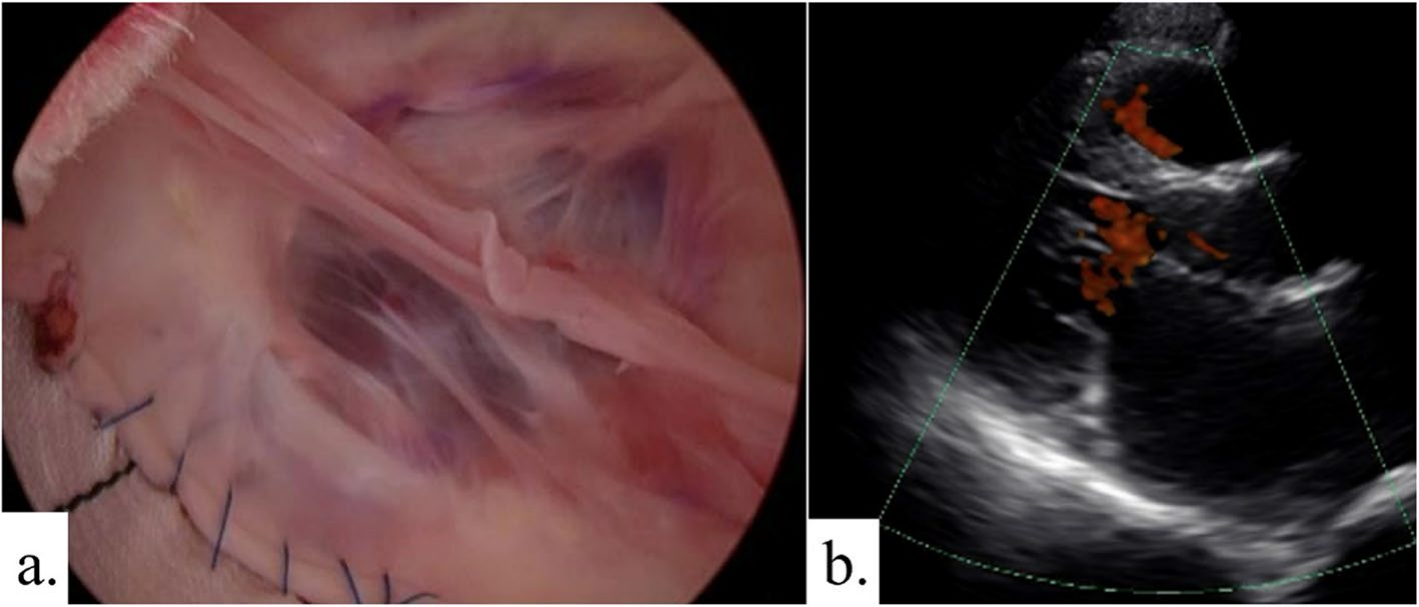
a Operative endoscopic view of aortic valve shows no prolapse and good valvular coaptation. b Postoperative transthoracic echocardiography showed no evidence of aortic regurgitation
